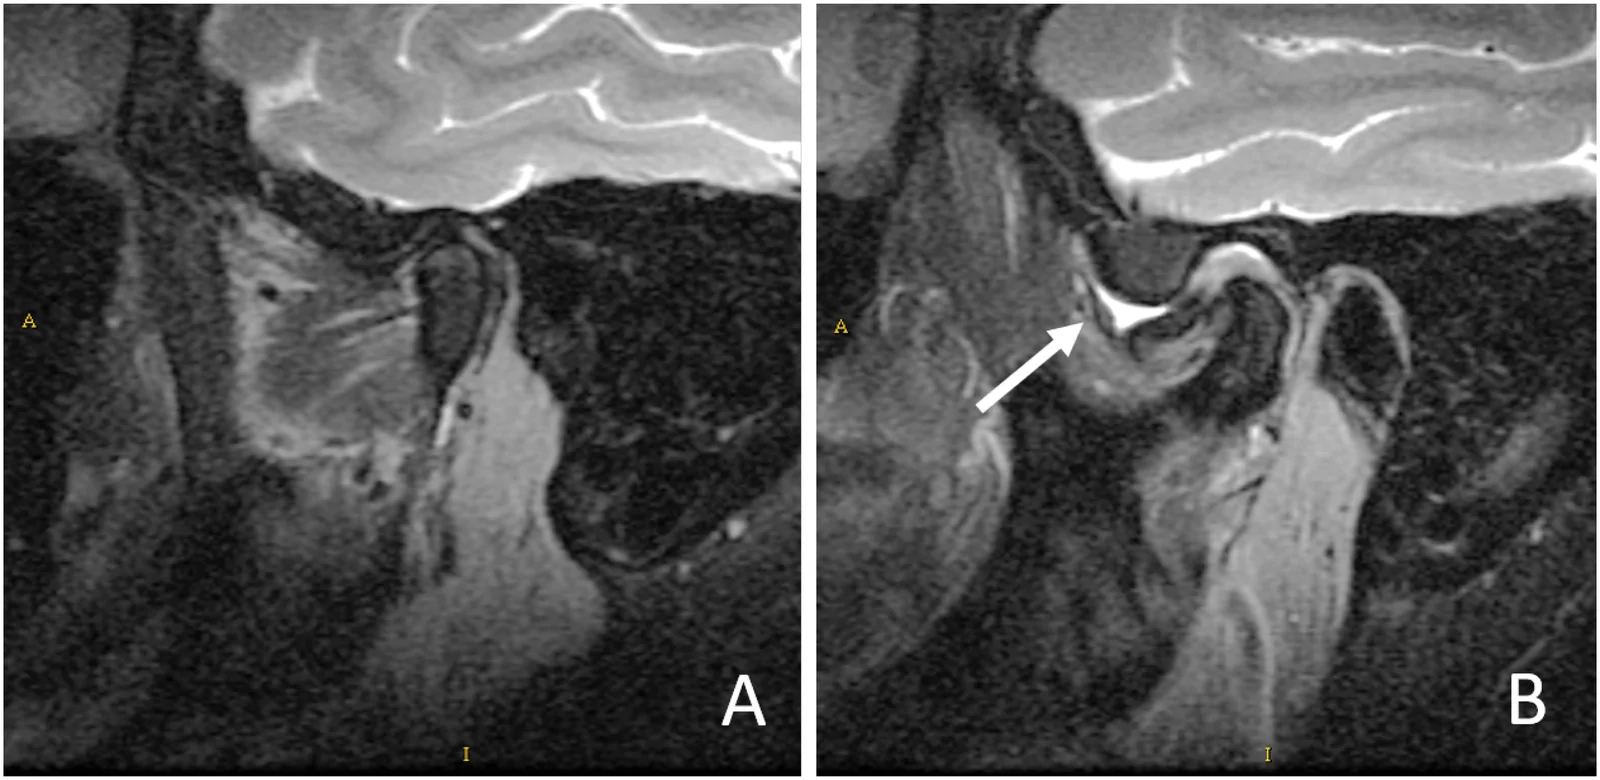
MRI sagittal images of the TMJ:. A) without effusion; B) with effusion.
